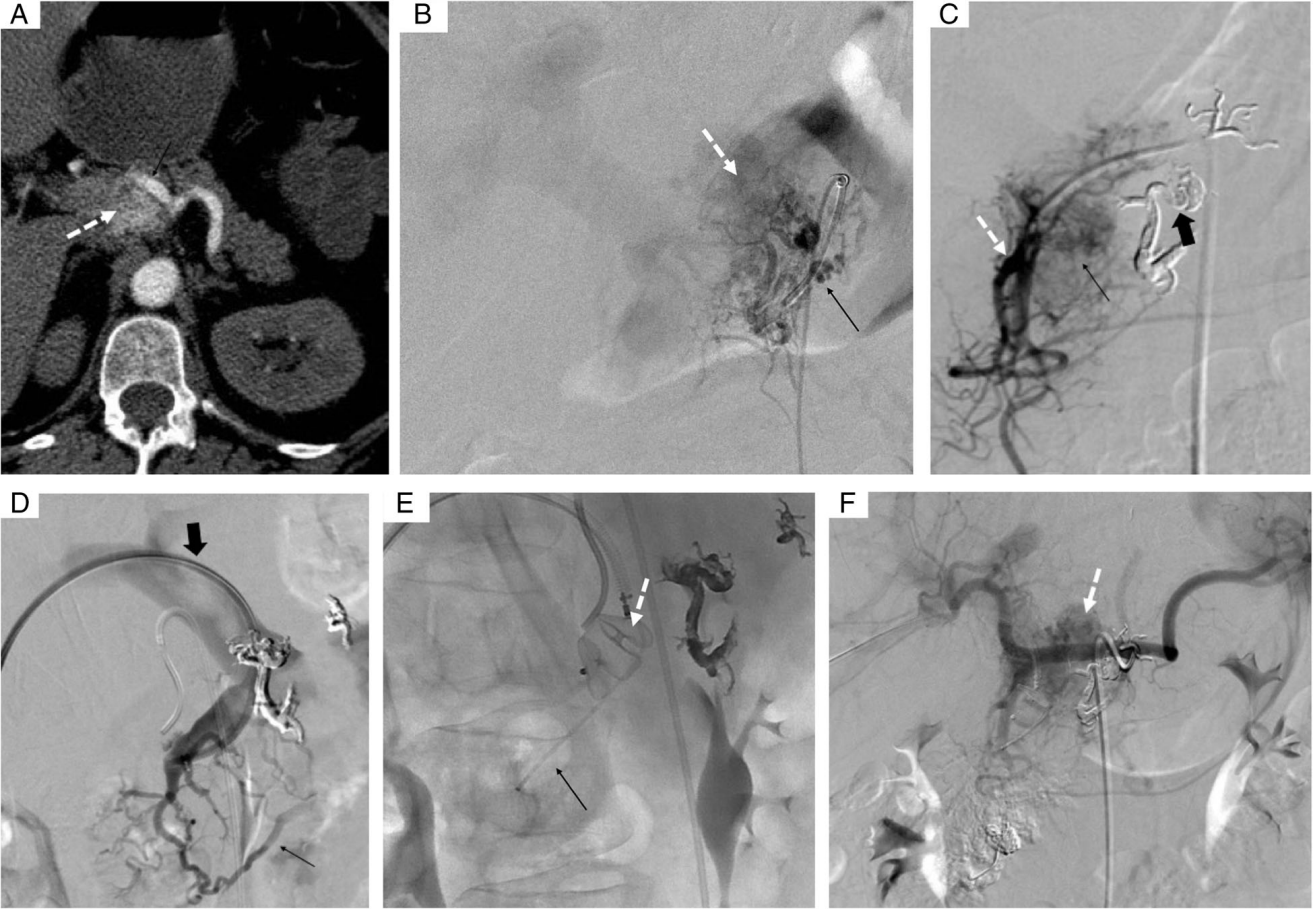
A 57 yo women with upper GI bleeding. A- CT scan showed a nidus in the pancreatic head (dotted arrow). B- Selective angiography of the pancreatic dorsal artery (black arrow) showing a type IIIB pancreatic AVM, with multiple feeding arteries draining in an aneurysmal vein (white arrow) both draining into the portal vein. C- Selective angiography of the gastroduodenal artery showing multiple arterial collaterals (black arrow), vascularized by multiples branches of the postero-superior and antero-superior pancreatico-duodenal arteries (white arrow). Embolization with Onyx® of the dorsal pancreatic artery (large black arrow). D- Portal venous access showed an enlarged pancreatic vein (black arrow) draining into the portal vein (big black arrow). E- Pressure cooker technique: proximal embolization of the draining gastroduodenal vein using a plug (white arrow), and then distal embolization with STS using a microcatheter distal to the Plug in order to reflux into the nidus (black arrow) of the AVM. F- Selective angiography of the celiac trunk showing a residual pancreatic AVM (arrow).
